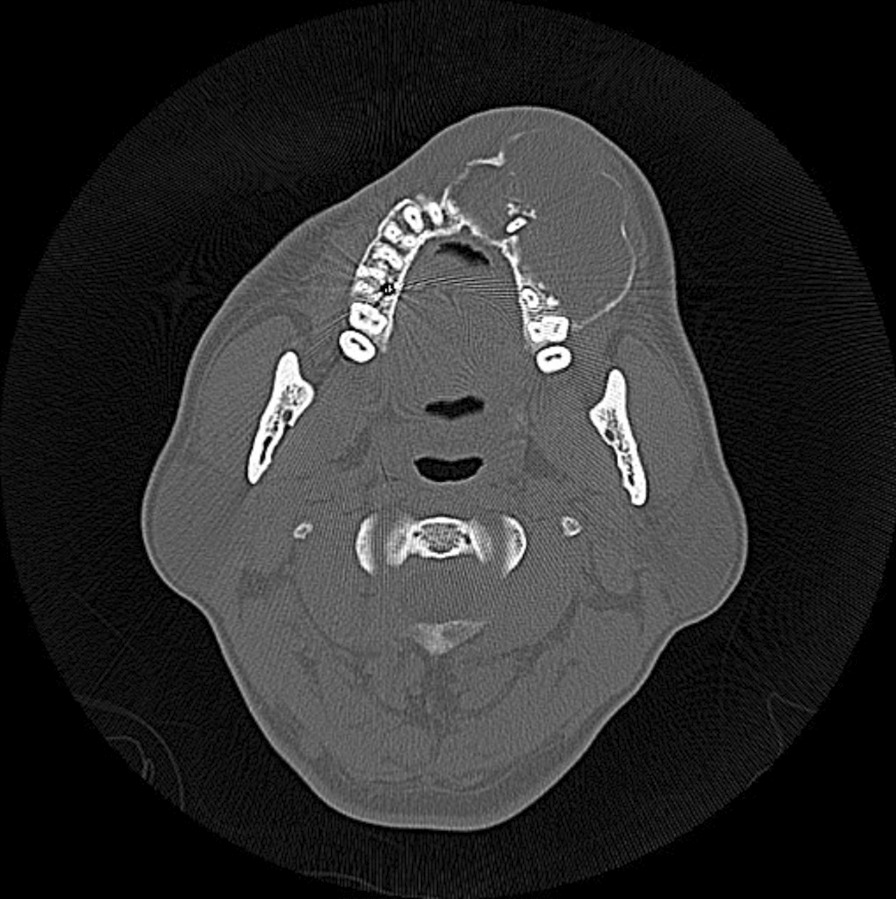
Computed tomography findings at the initial examination. A multilocular cyst-like lesion was observed in the right maxilla, and interspersed radiopacity in the interior of the solid lesion
